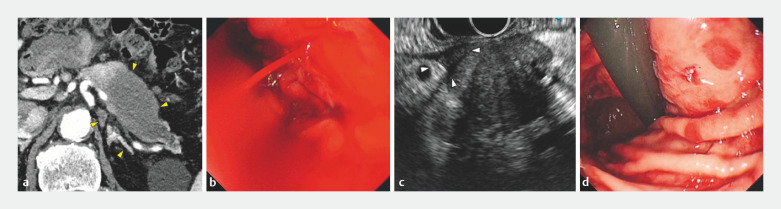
Bleeding and hemostasis during endoscopic ultrasound-guided tissue acquisition (EUS-TA).
